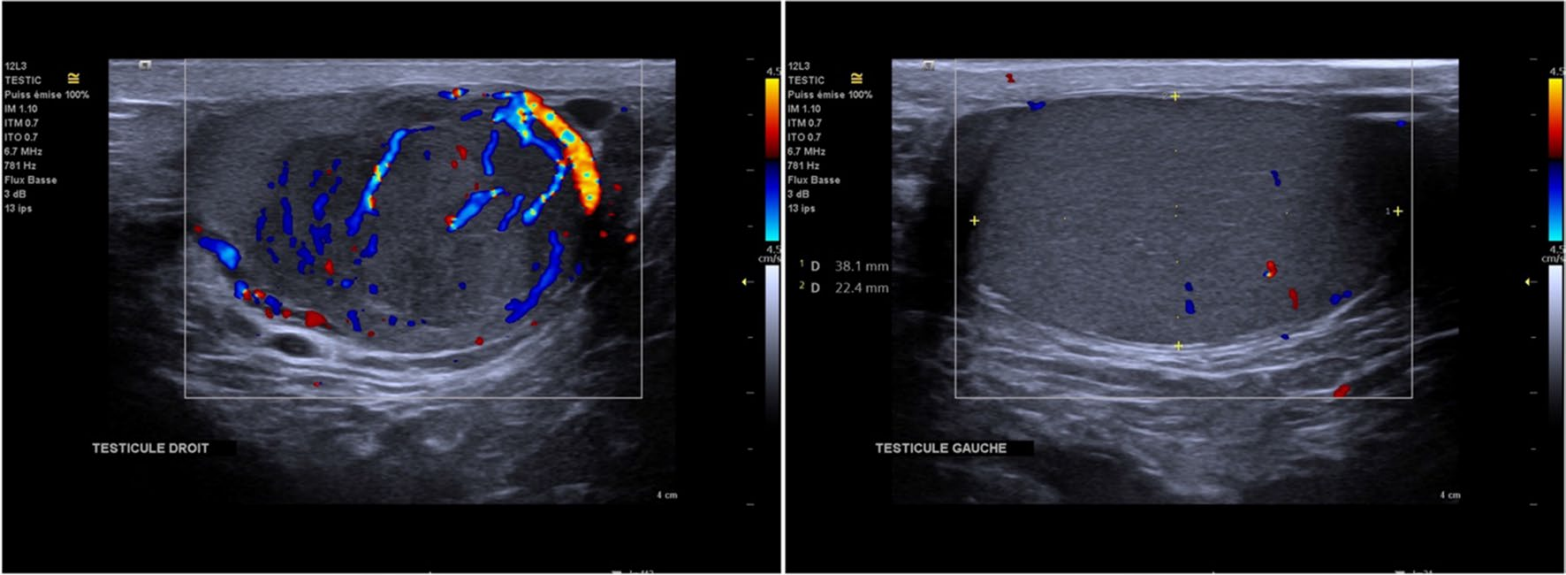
Testicular ultrasound shows right testicular vascularized mass with calcification (left) normal left testicle (right)
